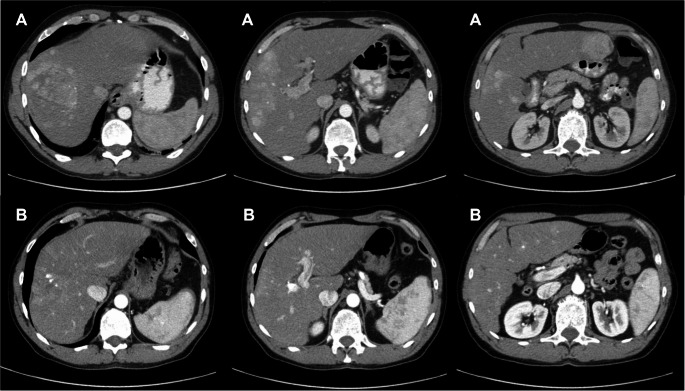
A: CT scan at baseline. B: CT scan after 93^h^ of Nivolumab infusion
